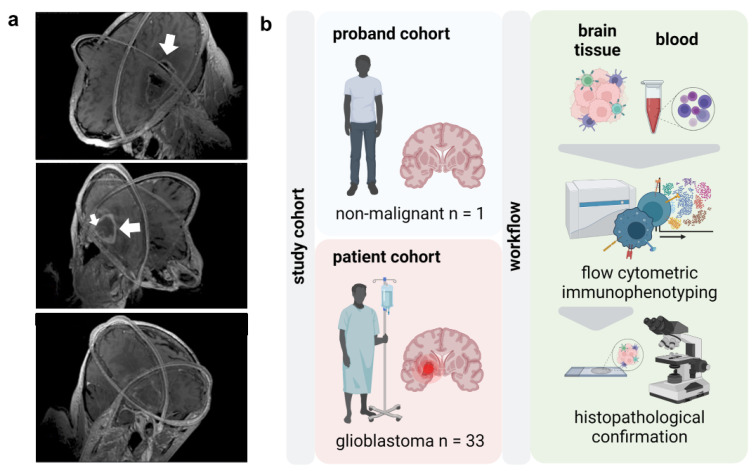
Study overview. (a) 3D visualizations of MRI scans of glioblastoma (white arrows) patients reconstructed from axial, sagittal, and coronal slices; (b) study overview.
